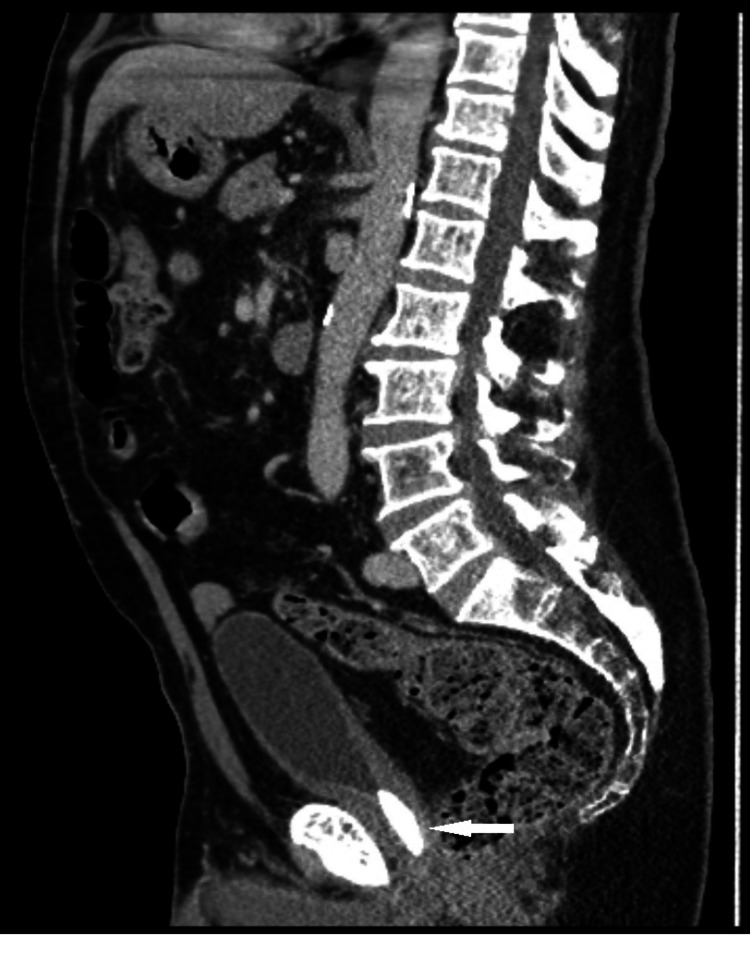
CT Abdomen saggittal view depicting large bladder stone at level of bladder neck
